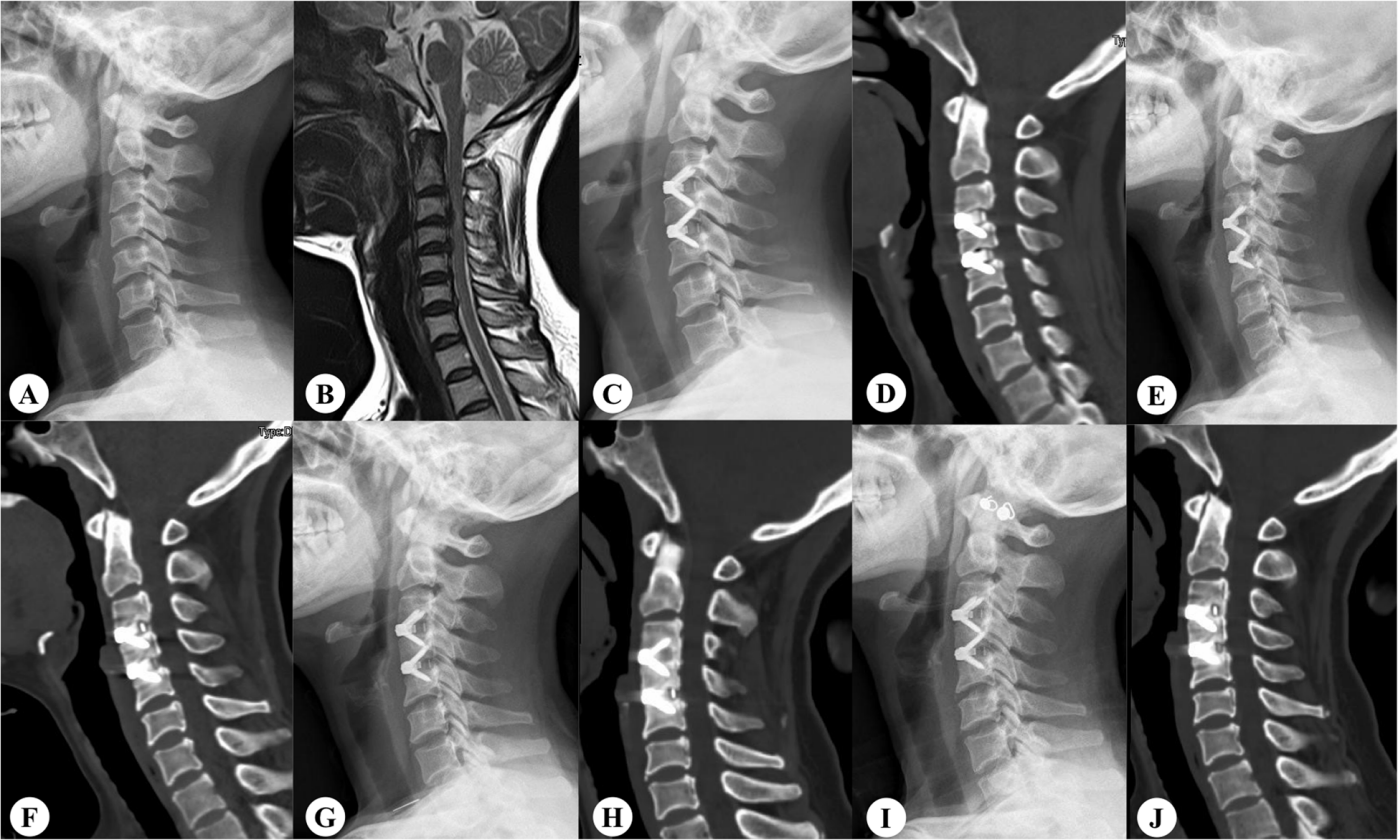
Radiographs of a 49-year-old woman. A, B Preoperative lateral radiograph showing degeneration and compression at the C3/4 and C4/5 levels. C, D Postoperative image showing two suitable prostheses placed at those levels. E, F Three-month postoperative lateral radiograph showing a growing bridge only at the cranial level. G, H Fusion of only the cranial level at 6 months postoperatively. I, J At 2 years postoperatively, fusion of only the cranial level is complete
